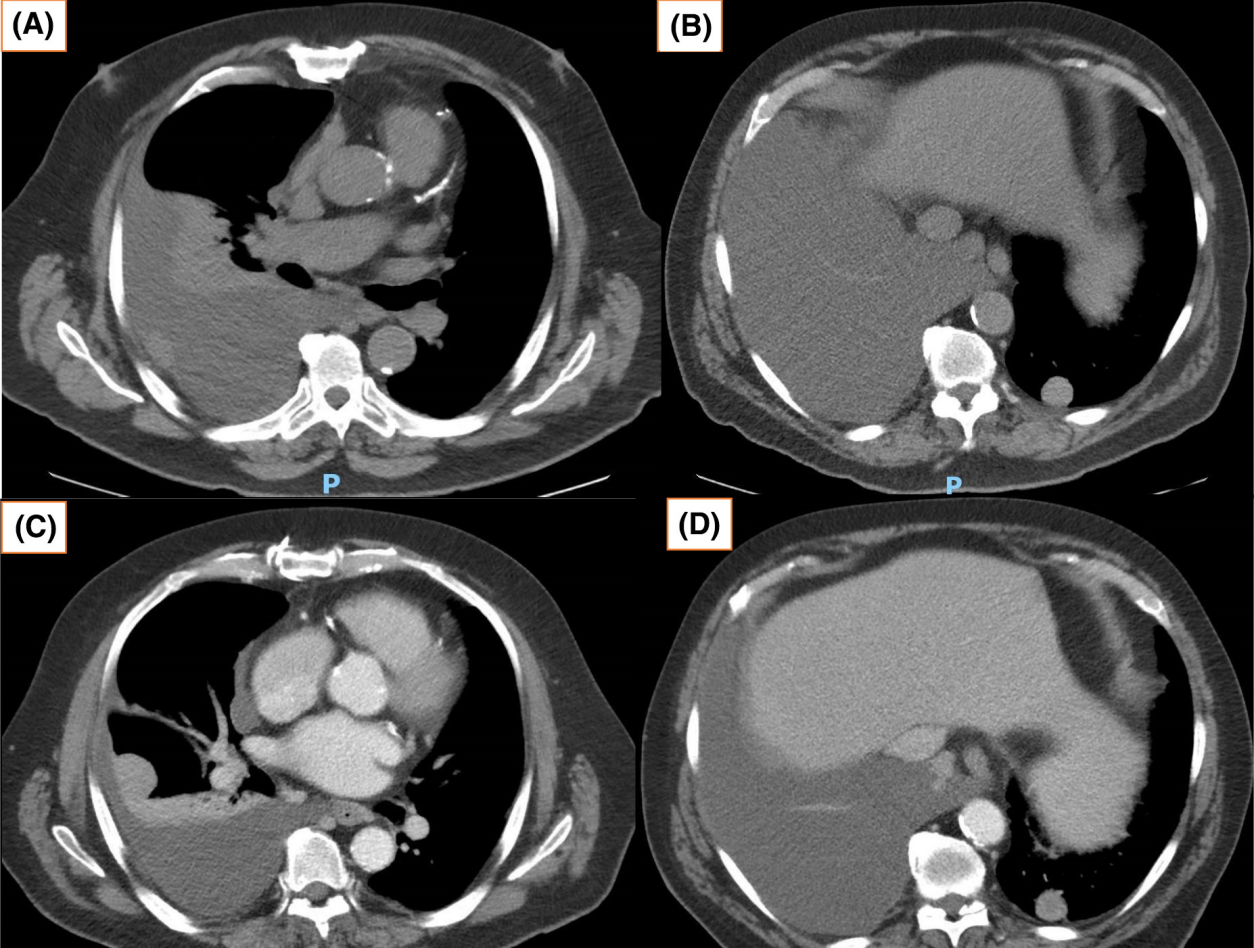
(A, B) July 2022, chest CT 1 week post C1D1 when the patient presented with increasing shortness of breath, demonstrating further disease progression. (C, D) July 2022 chest CT 1 week post drainage showing rapid accumulation of pleural effusion.
